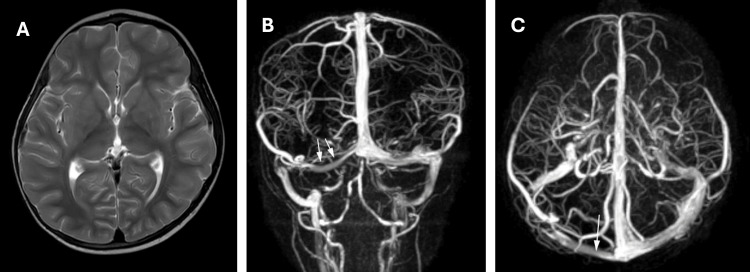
Brain magnetic resonance imaging with magnetic resonance venography on the second day of admission. (A) Axial T2-weighted image demonstrating fluid-fluid levels within the occipital horns of both lateral ventricles with dependent layering, consistent with proteinaceous or turbid cerebrospinal fluid rather than intraventricular hemorrhage. (B) Coronal magnetic resonance venography demonstrating irregular opacification of the right transverse and sigmoid sinuses (white arrows), consistent with venous sinus thrombosis. (C) Sagittal magnetic resonance venography demonstrating a linear filling defect with nodularity along the lateral aspect of the right transverse sinus (white arrow), consistent with acute dural venous sinus thrombosis.
